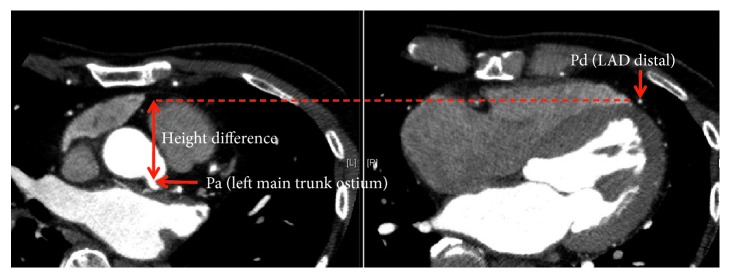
Height differences between Pa and Pd measurement by heart CT. Pa site was positioned at the ostium of the coronary artery on the CT image, and using side branches or tortuous parts as a landmark, Pd site was decided on the CT image. Two CT images matching the heights from the CT table were aligned, and the height differences between Pa and Pd were measured. CT, coronary tomography; Pa, mean aortic pressure; Pd, mean distal intracoronary pressure.
